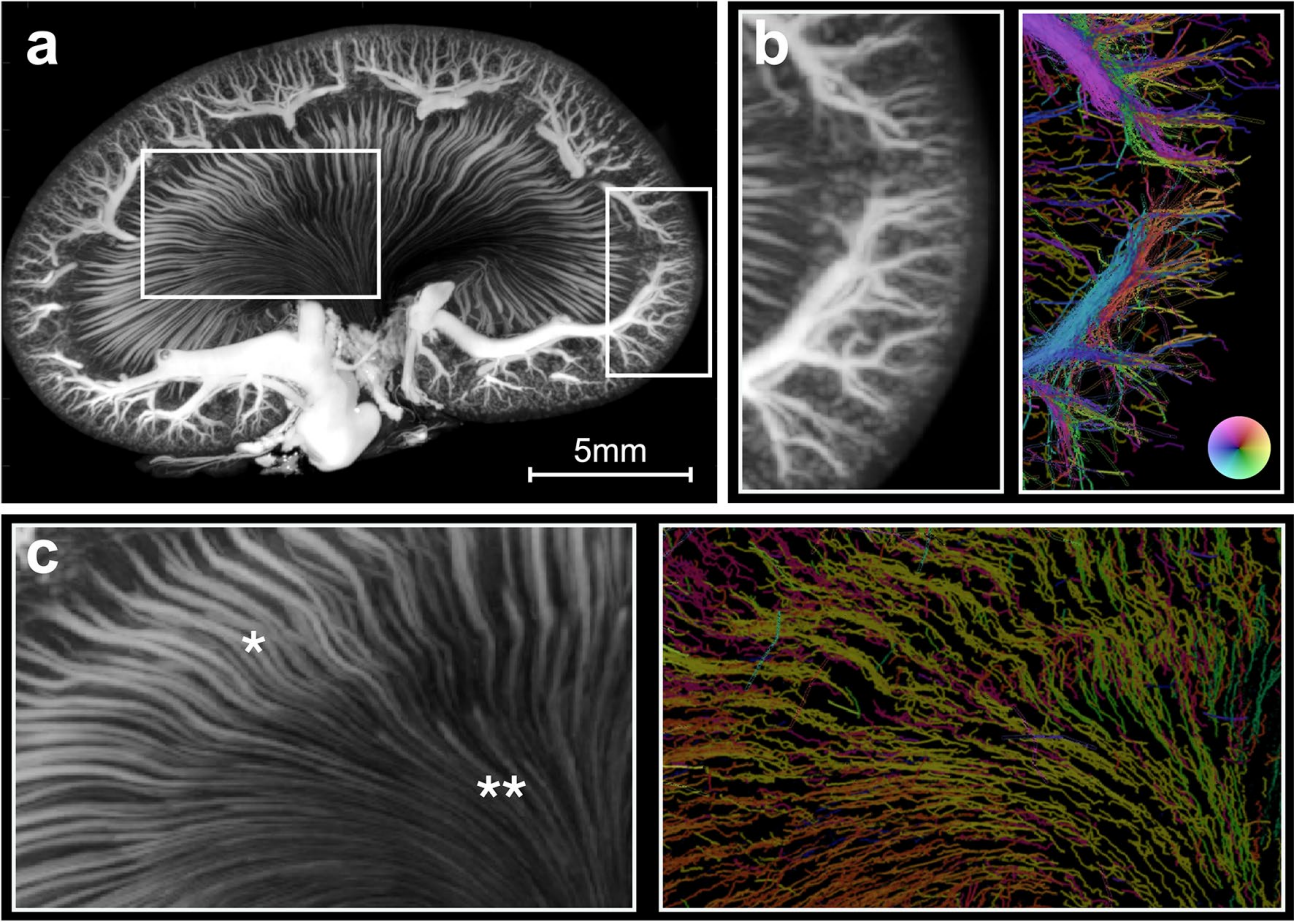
Visual comparison of the *μ*CT and microbubble track map. (a) *μ*CT maximum intensity projection in the super-resolution ultrasound image overlap of scan 1. The two marked regions are up-scaled and compared with the corresponding unfiltered microbubble track map in (b) and (c) (for visualization, the color transparency in the microbubble track map is scaled by the intensity map). (b) Shows a part of the renal cortex. (c) Shows a part of the renal medulla with the vasa recta. Notice how the color wheel in the microbubble track map allows separation of arteries and veins according to flow direction, e.g*.*, in (c) with the descending (orange/yellow/green) and ascending (purple/blue) vasa recta of the medulla. * marks outer medulla,  marks inner medulla.
